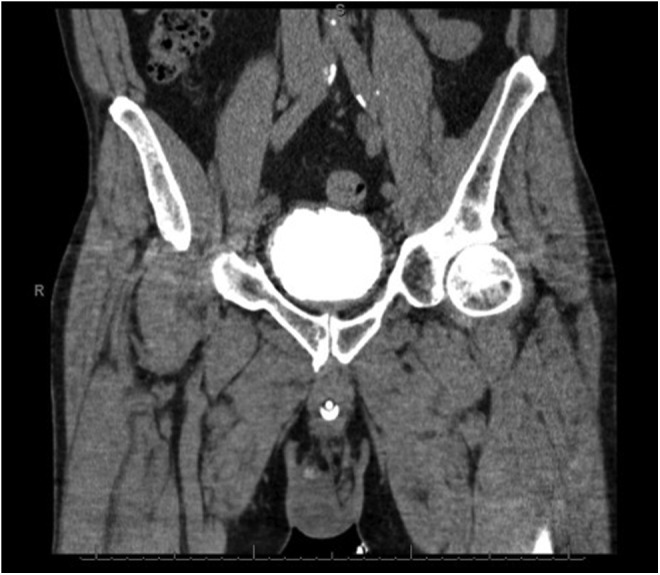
CT cystogram reveals no evidence of leak. Previous perivesicular fluid has resolved.
